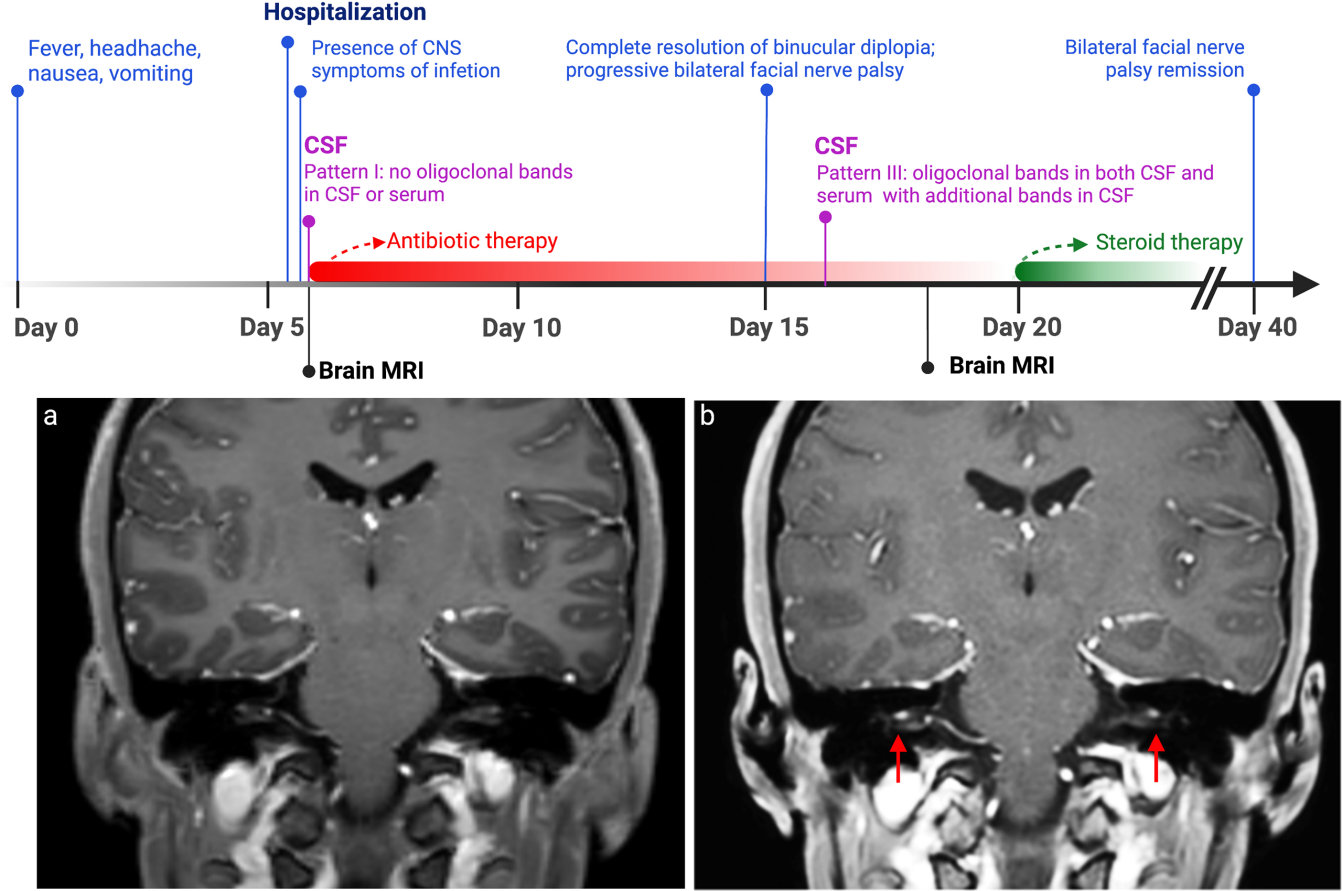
Disease timeline. In this timeline are displayed clinical (shown in blue) and CSF (in purple) changes in relation to antibiotic course (in red) and steroid therapy (in green). On the bottom, MRI T1-weighted imaging with gadolinium contrast performed after 18 days from clinical onset (A) demonstrated bilateral pathologically relevant gadolinium enhancement in VII and VIII cranial nerves’ intrameatal tract bilaterally (red arrows), which was not present in the first MRI (B). CSF, cerebrospinal fluid.
